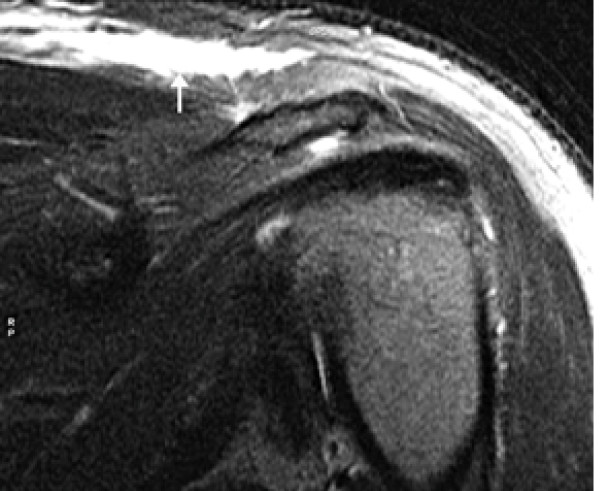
Coronal oblique fat-suppressed post contrast MRI left shoulder (TR/TE 5670/75).
